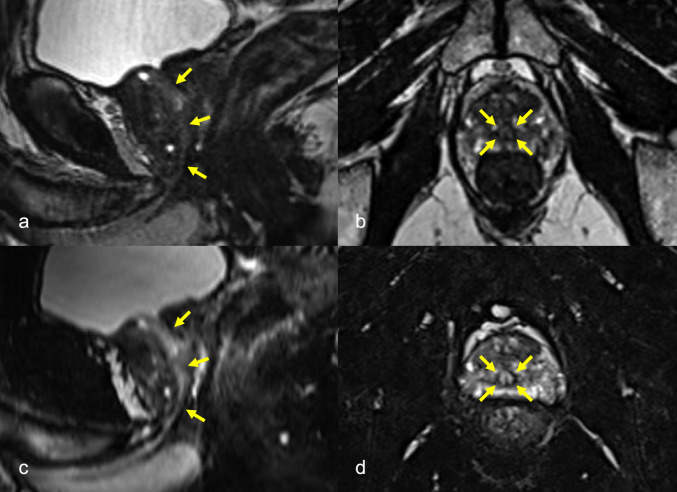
Images of a 76-year-old male in Case 1. The prostatic urethra is identified as a high-intensity tract (arrows) in the conventional 3D T2-weighted sagittal (a) and transverse (b) images, and it is more clearly identified in the proposed fat-suppressed 3D T2-weighted sagittal (c) and transverse (d) images
